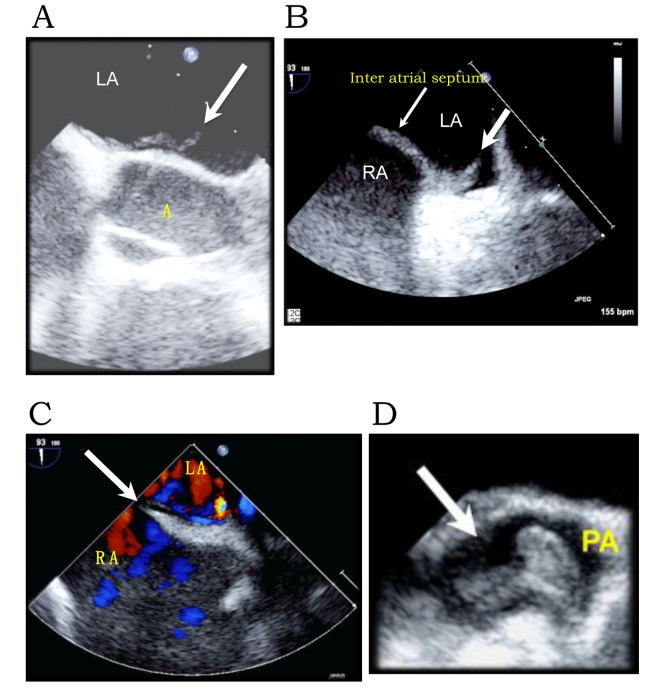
Transesophageal ECHO. (A) Serpiginous thrombus in the LA. (B) Thrombus straddling across the PFO (broad arrow). (C) Color Doppler demonstrating flow across the inter-atrial septum via the PFO (broad arrow). (D) Embolus in the PA (arrow).
